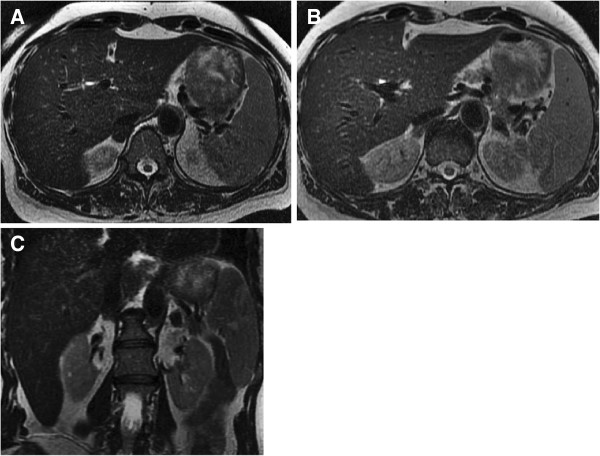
Abdominal magnetic resonance imaging obtained 3.5 months after surgery. Axial (A, B) and coronal (C) T2-weighted images showing a decrease in size and a change of signal of the bilateral adrenal hematomas. The lesions now have homogeneous low signal, due to haemosiderin.
